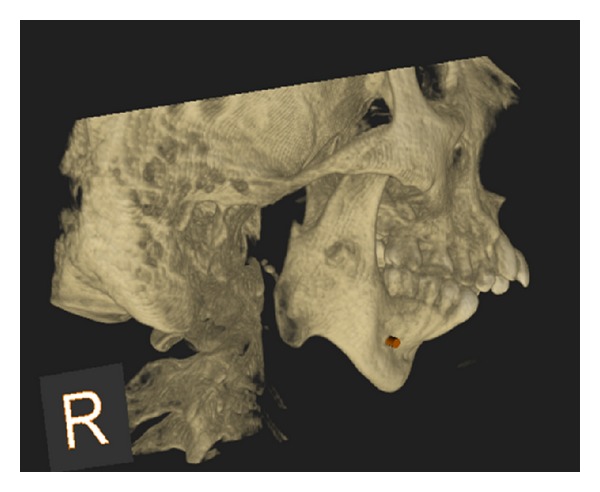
3D CBCT right lateral shows absence of glenoid fossa and complete absence of the condyle.
